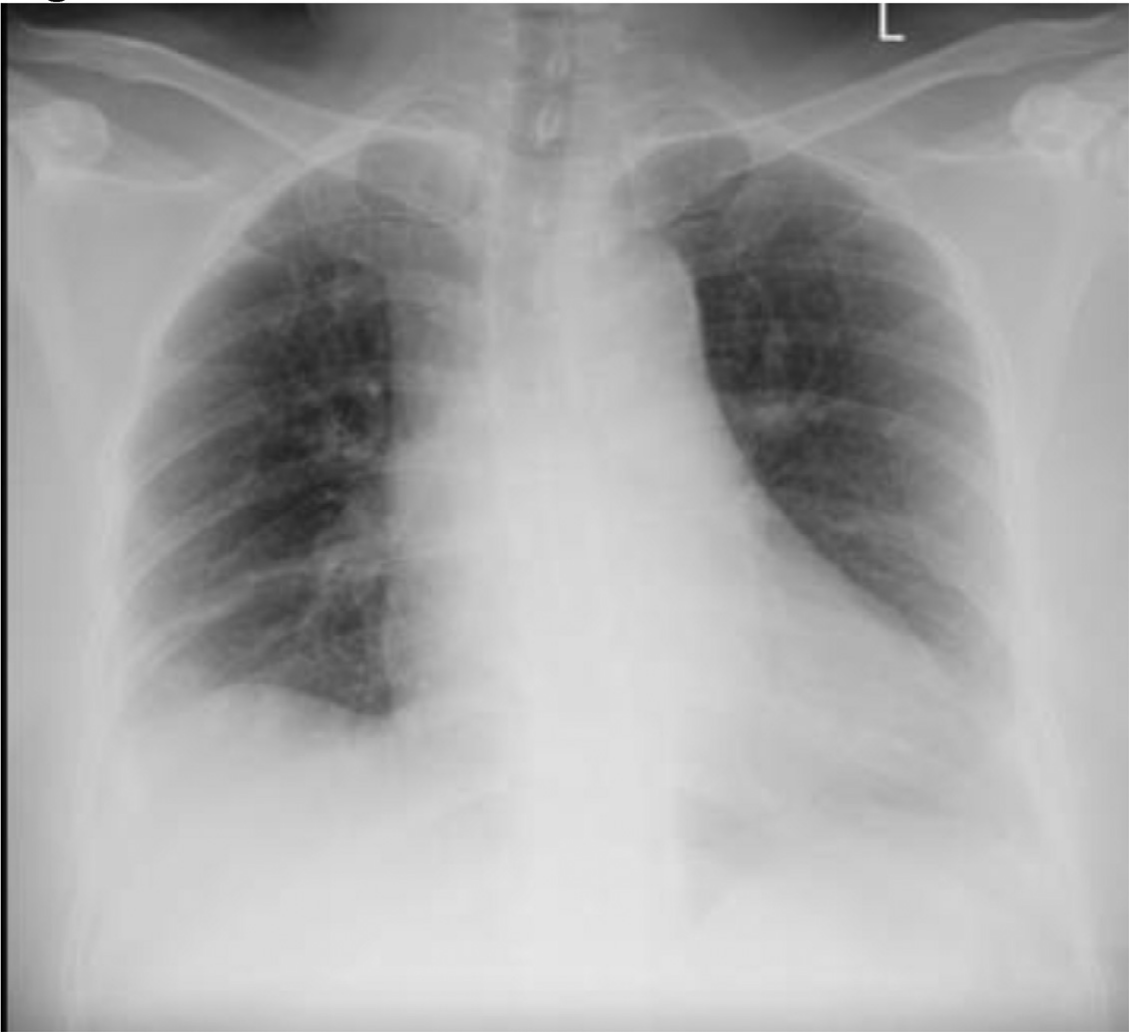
Chest X-ray showed cardiomegaly with atherosclerosis aorta, with no lung abnormalities.
